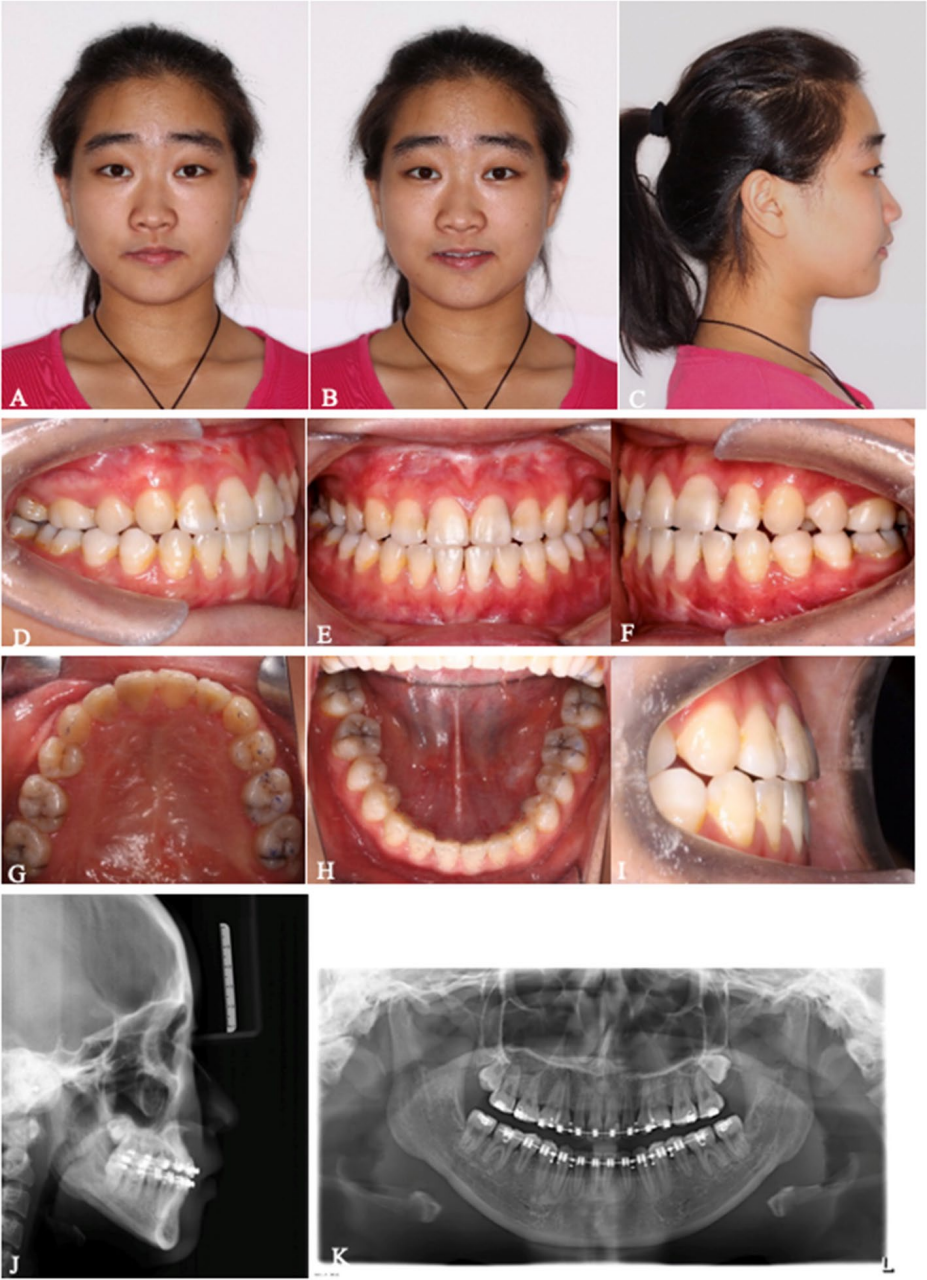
A-K Photographs, intraoral view, lateral cephalometric and panoramic radiographs after orthognathic surgery and postoperative orthodontic treatment of #1. The titanium plates were removed 8 months after BSSRO. All appliances were removed and the final X-rays were taken 12 months after BSSRO
